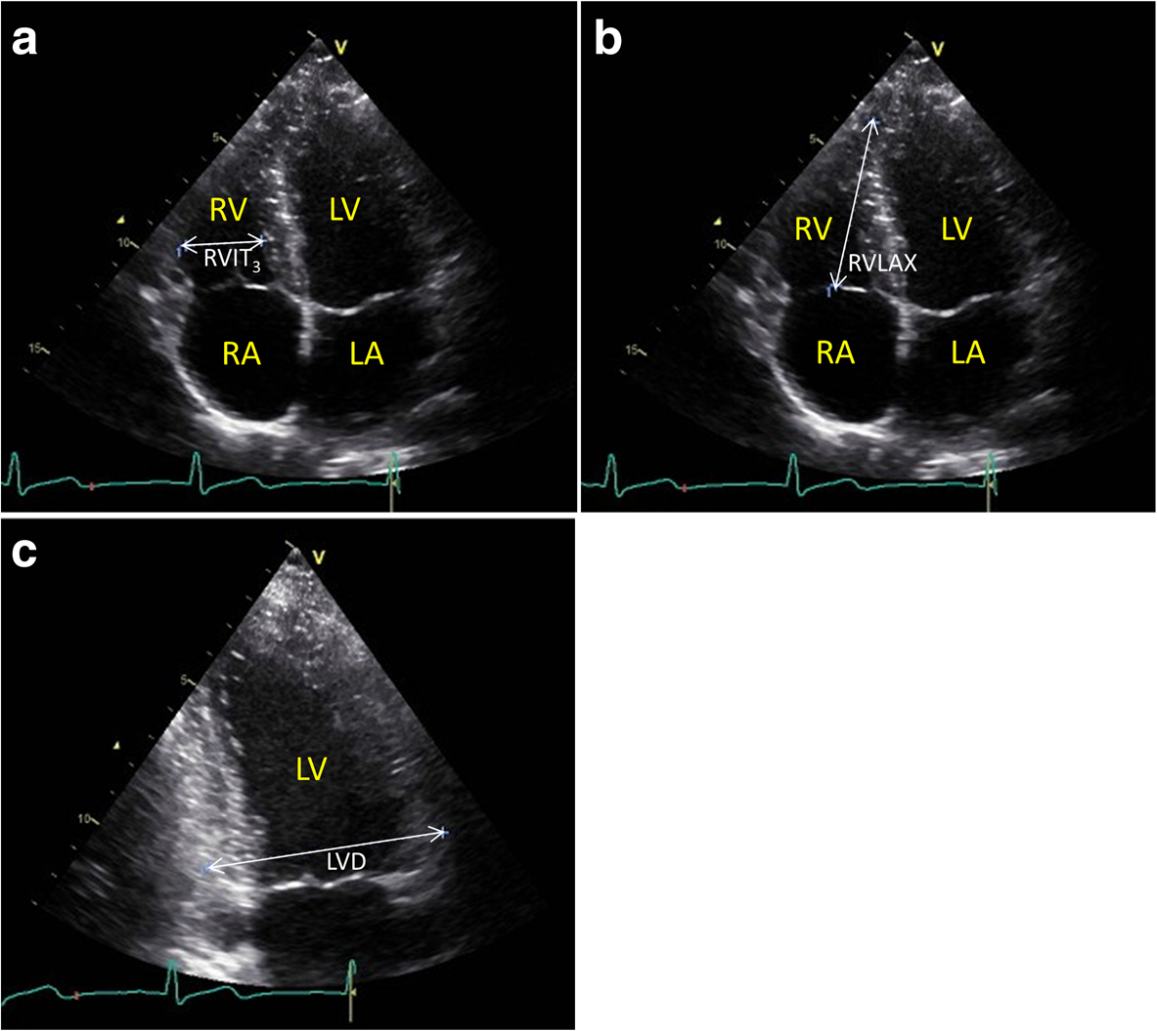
Transthoracic echocardiography distances. Images showing the transthoracic echocardiography distances for a healthy 33 year old male subject in a) and b) apical 4CH view and in c) apical 2CH view. LA = left atrium, LV = left ventricle, LVD = left ventricular diameter, RA = right atrium, RV = right ventricle, RVIT_3_ = right ventricular inflow tract, RVLAX = right ventricular long axis
